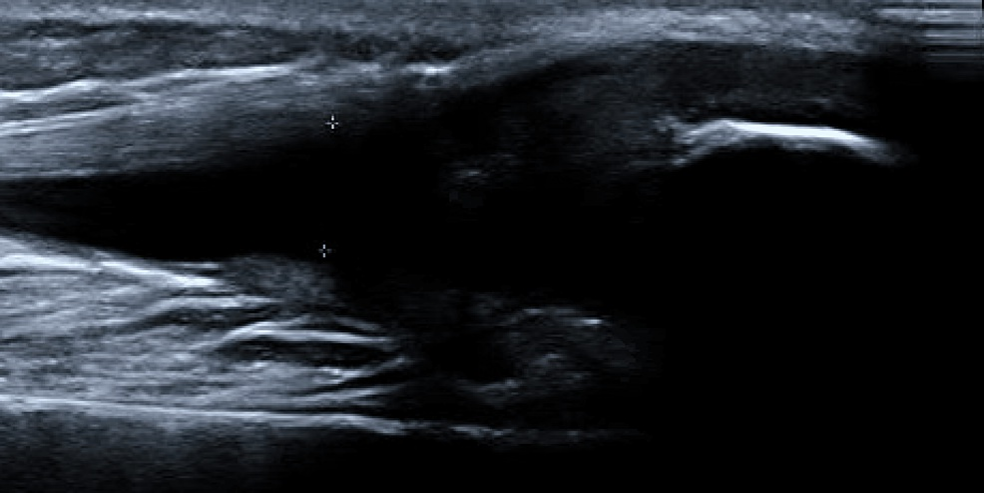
Long segmented view of the left knee US. Long segmented view of the left knee US with the pocket of fluid near the patella, marked by plus signs. US: ultrasound.
